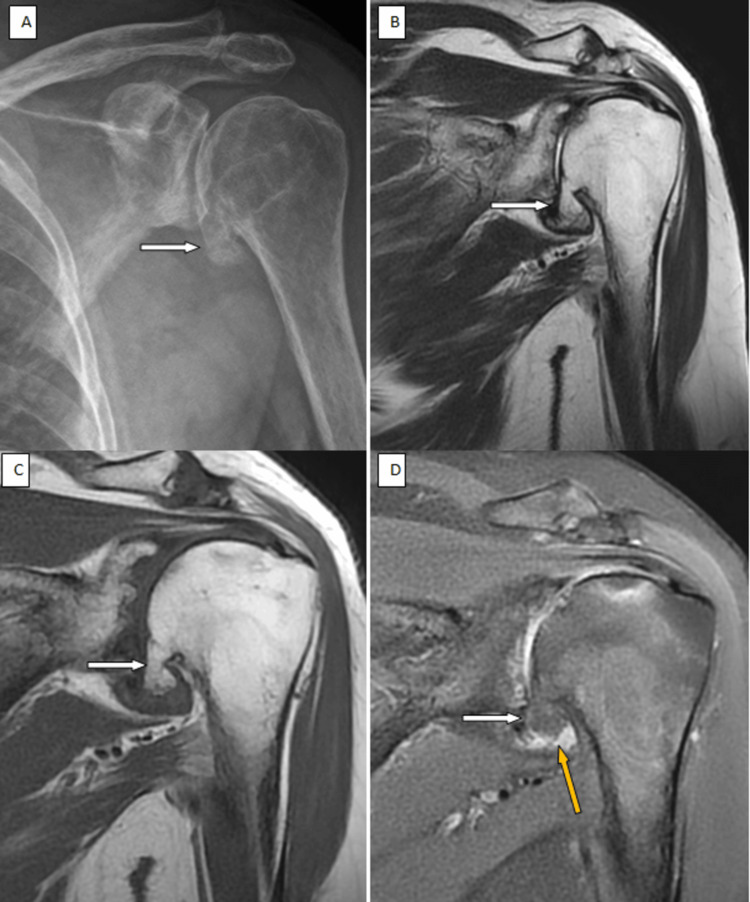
A: X-ray AP view of the left shoulder showing a hanging bony lesion from the humerus (white arrow). B: T2WI and C: T1WI showing an intracapsular bony lesion arising from the posteromedial part of the left humeral head in continuity with the cortex and medulla of the humerus, suggestive of intracapsular osteochondroma (white arrow). D: STIR coronal image notes that the joint capsule is distended, with small effusion (orange arrow). There is no significant thickening of the overlying cartilaginous cap (3 mm). AP: anteroposterior; T2WI: T2-weighted imaging; T1WI: T1-weighted imaging; STIR: short tau inversion recovery sequence
